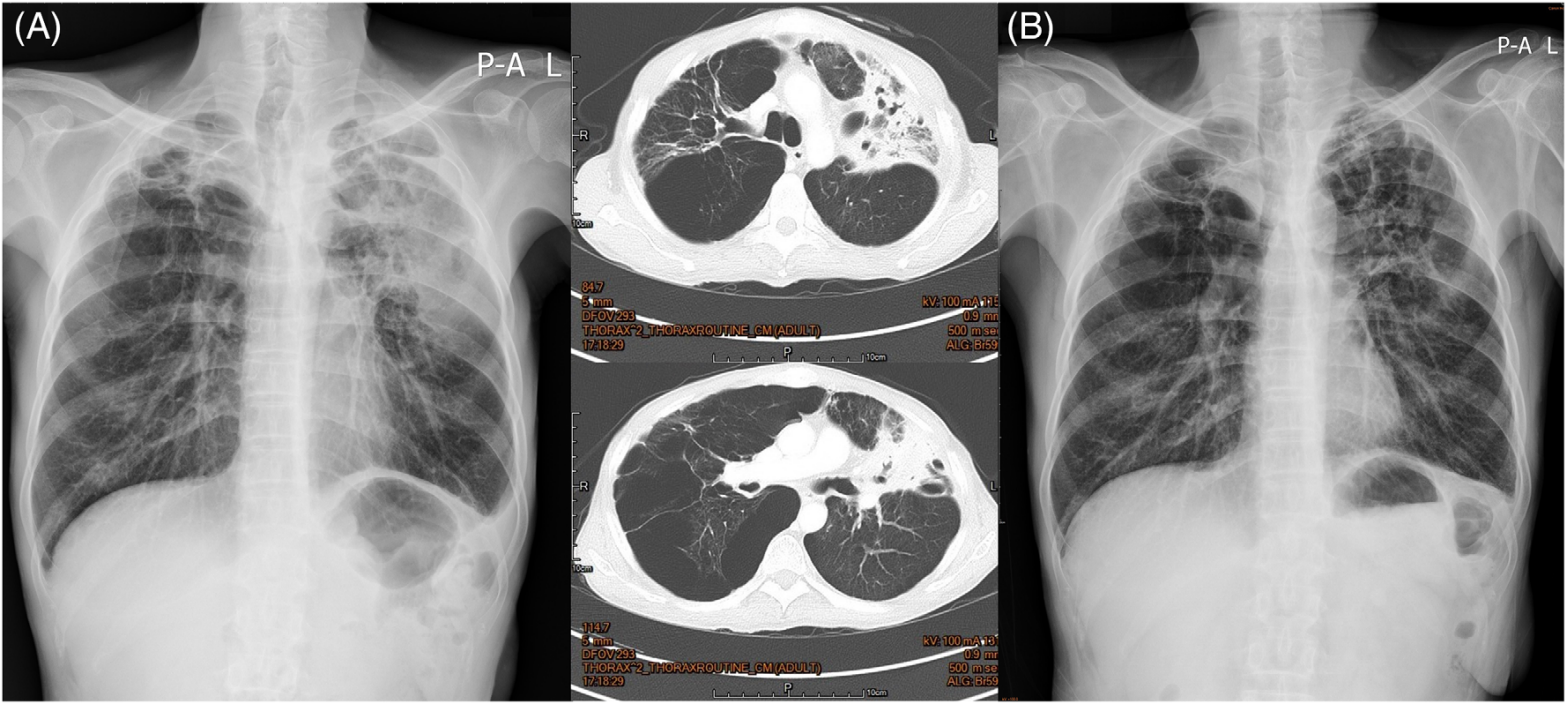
(A) Chest x‐ray (CXR) and computed tomography (CT) imagines showing an emphysematous lung with significant bullae and bilateral upper lung consolidation, which was more severe on the left side (CXR and CT taken in 2021/12). (B) CXR showing stationary emphysematous lung with significant bullae but with bilateral upper lung consolidation much improved compared to (A) (CXR taken in 2022/2).
